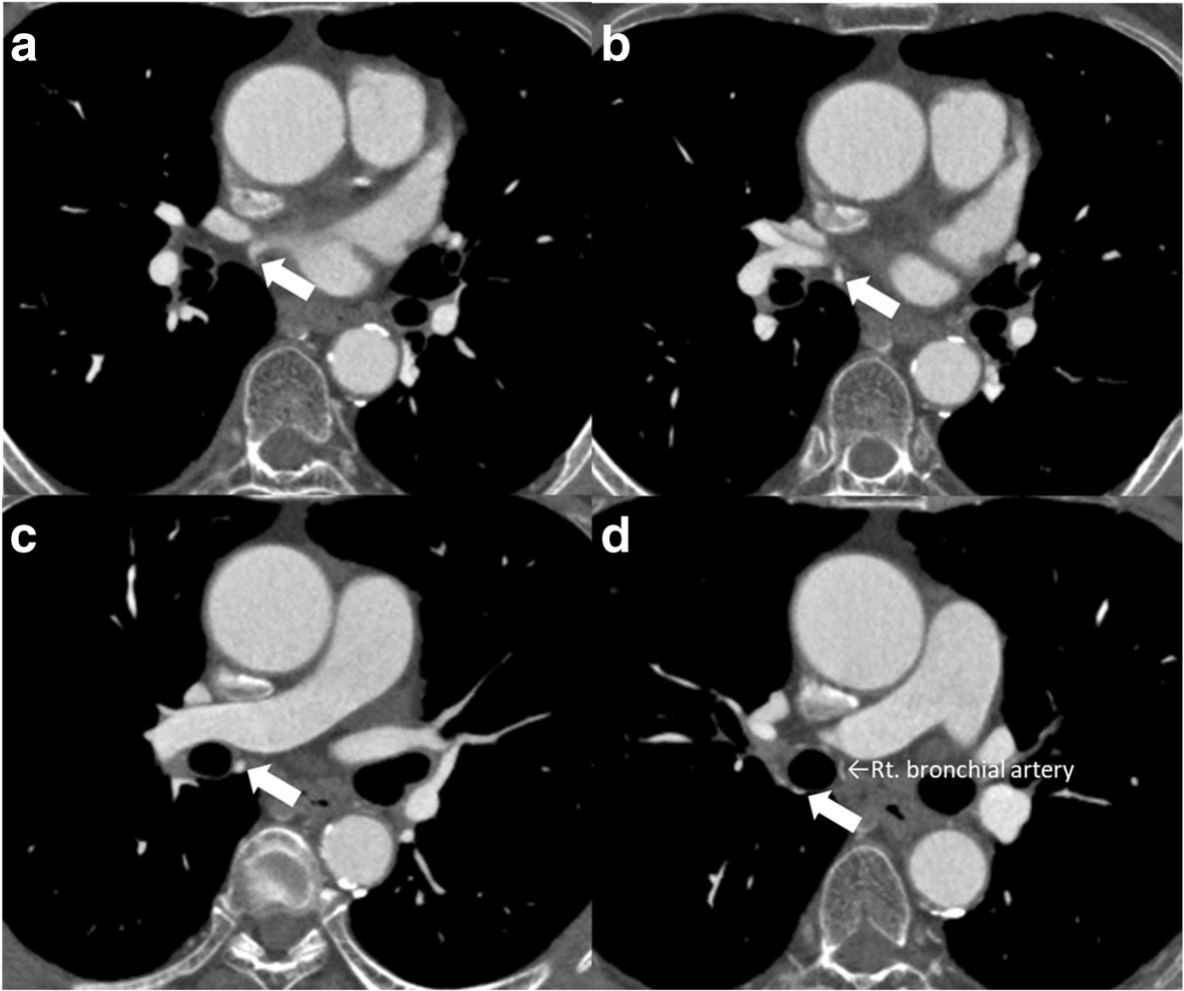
CT image of the course of the anomalous V2. a Anomalous V2 draining into the RSPV. b–d Anomalous V2 running behind the intermediate bronchus
